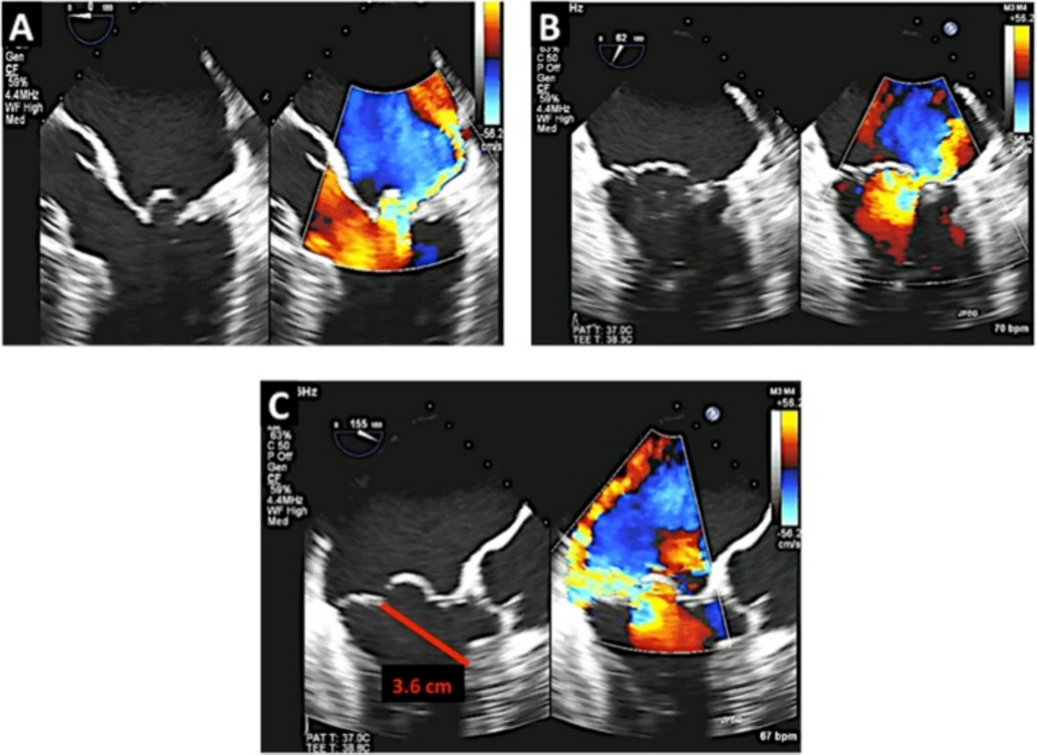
2D and color Doppler assessment of Type II MR. A: 2D TEE at 0 degrees demonstrates severe posteriorly directed MR due to a flail A2 scallop. B: TEE at the bicommisural view again demonstrates the origin of the MR. C: The origin of the MR is between the A2/P2 scallops. The coaptation-septum distance is also demonstrated.
